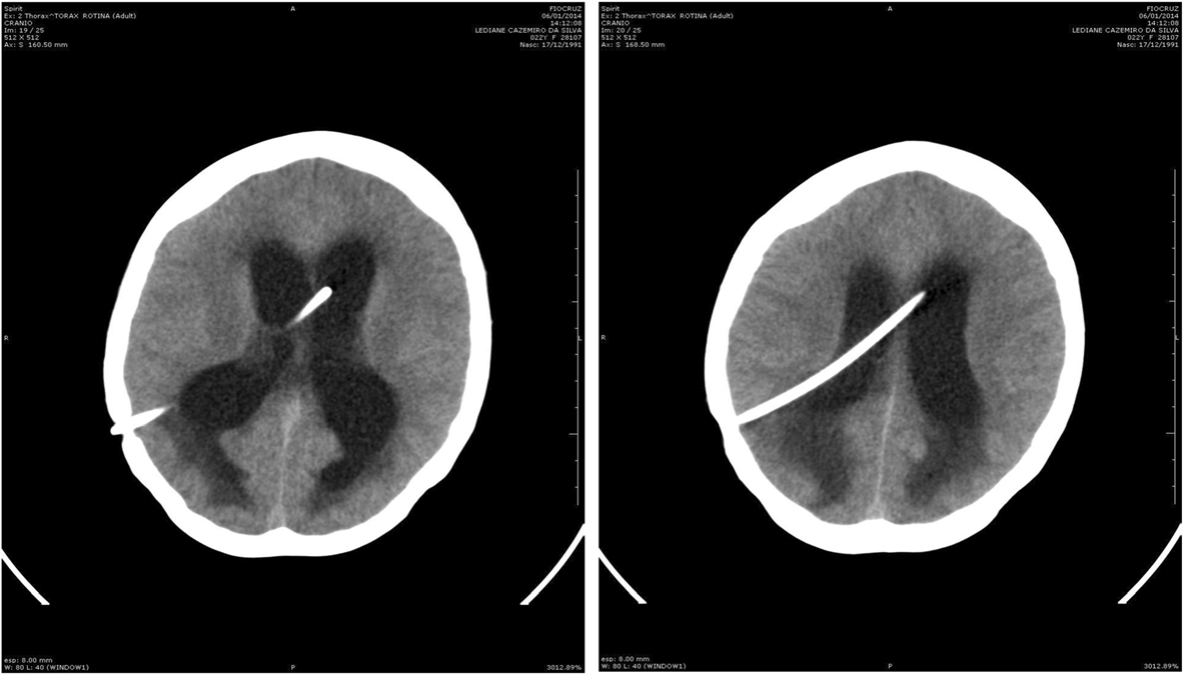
Brain CT scan on January 2014, showing intraventricular shunt in place and hydrocephalus.
